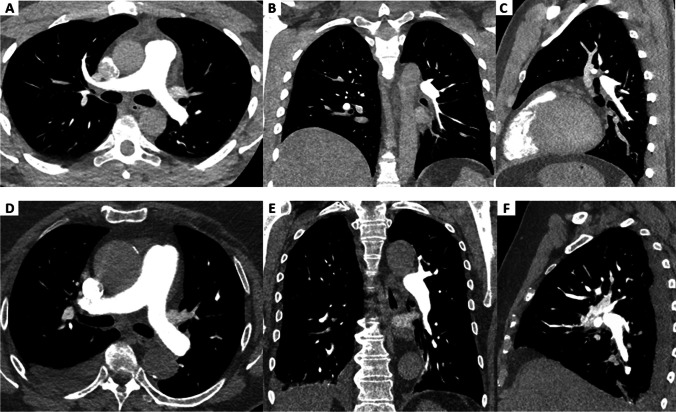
Comparison of the two protocols. Comparison of the two high-pitch CTPA (A–C) at 100 kVp of a 38-year-old male (BMI 28.7 kg/m^2^) and standard CTPA at 100 kVp of a 68-year-old female patient (BMI 30.1 kg/m.^2^) in axial (A, D), coronal (B, E), and sagittal (C, F) view. Subjective and objective image quality were excellent for both studies (mean opacification of the pulmonary arteries: 375.0 ± 27.2 HU; 441.3 ± 36.7 HU). Radiation dose for high-pitch CTPA was lower comparted to standard CTPA (DLP: 89.6 mGy·cm; 135.0 mGy·cm, CTDI_vol_: 3.2 mGy/cm; 4.9 mGy/cm and ED: 1.3 mSv/mGy·cm; 1.9 mSv/mGy·cm). BMI, body mass index; CTDI_vol_, volume CT dose index; CTPA, computed tomography pulmonary angiography; DLP, dose-length product; ED, effective dose, calculated as DLP × conversion factor of thorax (*k* = 0.014 [34])
